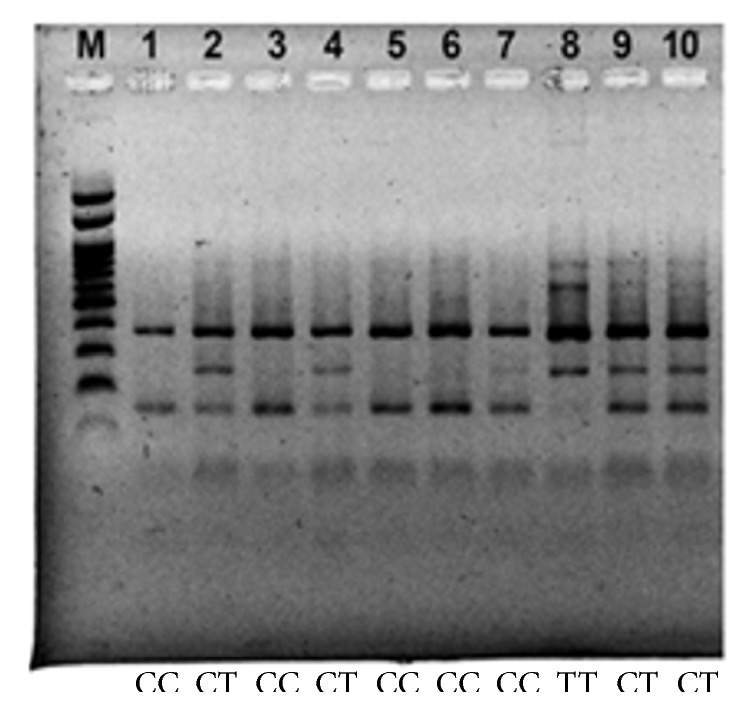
Renin gene C-5312T amplification. Photos of electrophoresis results (PCR product of 10 hypertensive patients), with an ethidium bromide dye on agarose 1.5%. From the left, image (M) is marker, and wells 1 to 10 are a band of renin gene polymorphism with genotypes CC, CT, and TT.
